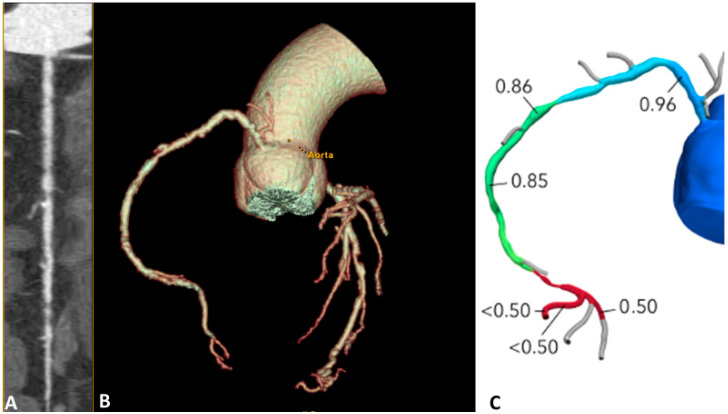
CCTA and FFR_CT_ combined evaluation in a 65-year-old patient complaining of angina. (A) The study of the right coronary artery reveals multiple plaques, with stenosis ranging visually from severe to moderate. (B) 3D volume rendering reconstruction. (C) FFR values are pathological only in the mid-distal segment, indicating hemodynamically significant stenoses at this level. CCTA: Coronary Computed Tomography Angiography; FFRCT: Fractional Flow Reserve Coronary Tomography.
